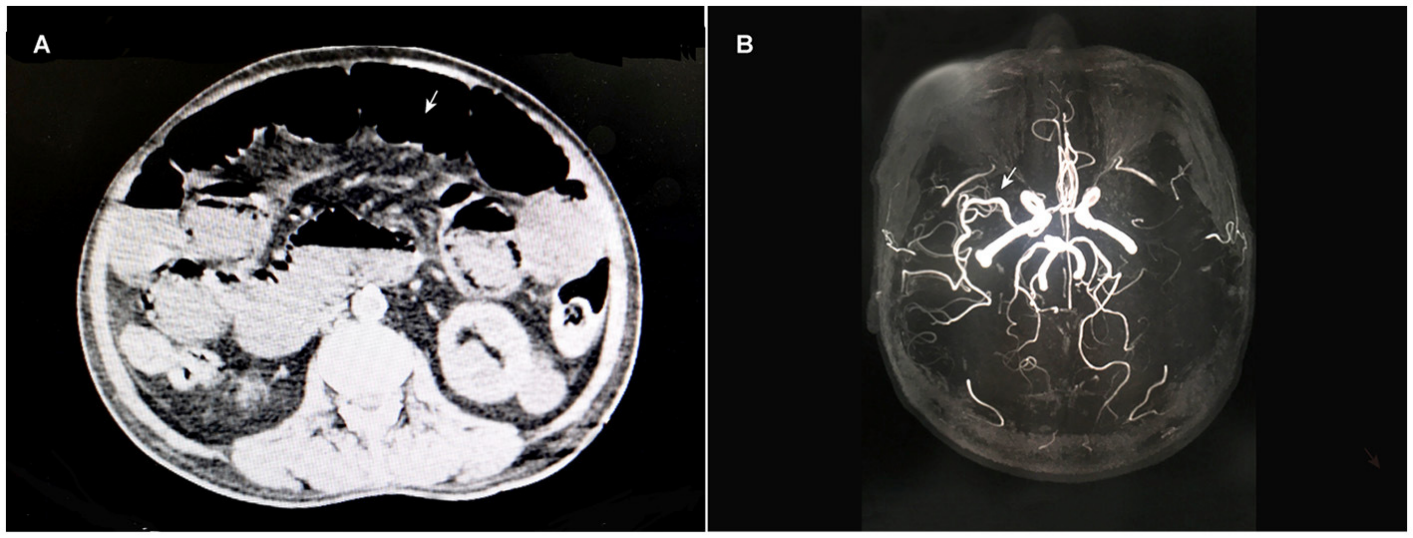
Abdominal CT scans (A) showed rectal cancer and an intestinal obstruction. The cerebral MRA image (B) showed that the left middle cerebral artery was invisible, while the right was normal.
